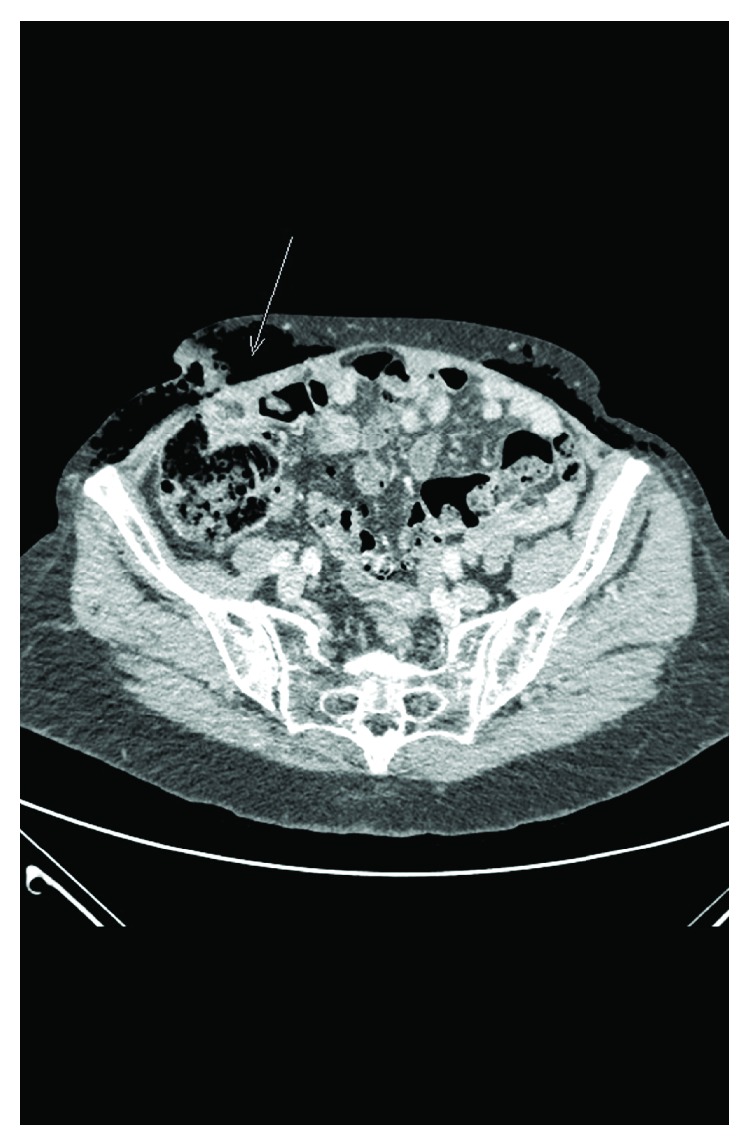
CT findings of the first case. The arrow indicates the extraperitoneal perforation.
